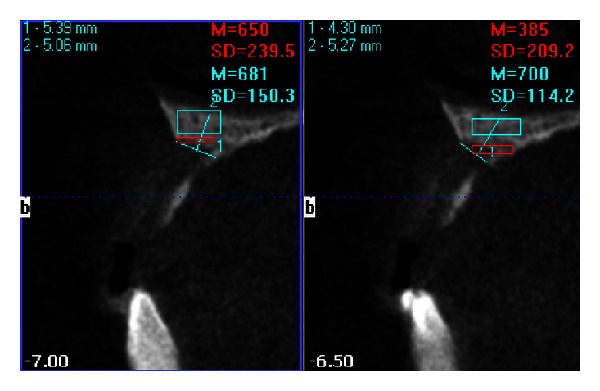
Transplanar images of of edentulous area on CBCT. Gross deficiency in dimensions of alveolar ridge is evident.
